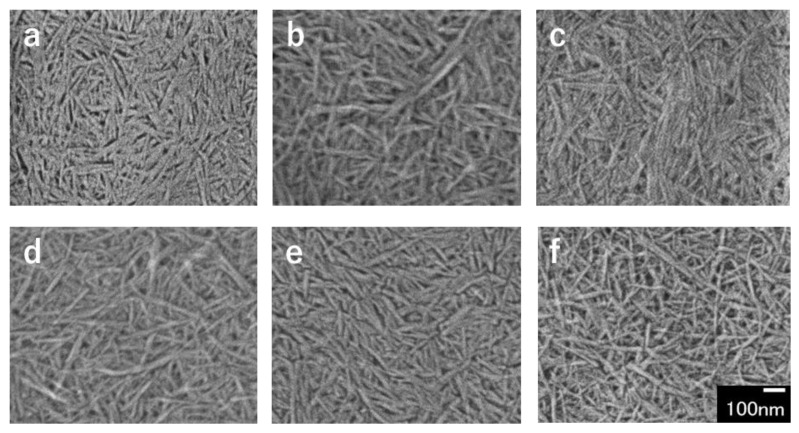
FE-SEM images of partially deacetylated chitin nanofibers with ultrasonication time of (a) 5, (b) 10, (c) 15, (d) 20, (e) 25, and (f) 30 min.
